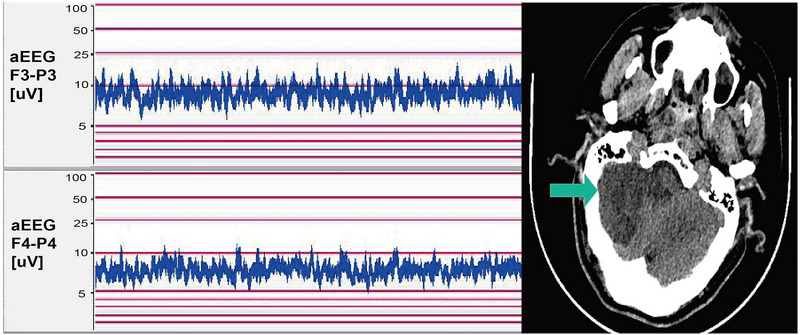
Post‐rewarming QEEG changes and corresponding brain CT findings. Following rewarming, QEEG initially showed symmetrical aEEG readings, but a progressive decrease in the right aEEG was observed. Postoperative brain CT later confirmed a large cerebral infarction in the right hemisphere. F3‐P3 and F4‐P4 corresponded to the left and right hemispheres. aEEG, amplitude‐integrated electroencephalography; QEEG, quantitative electroencephalography.
